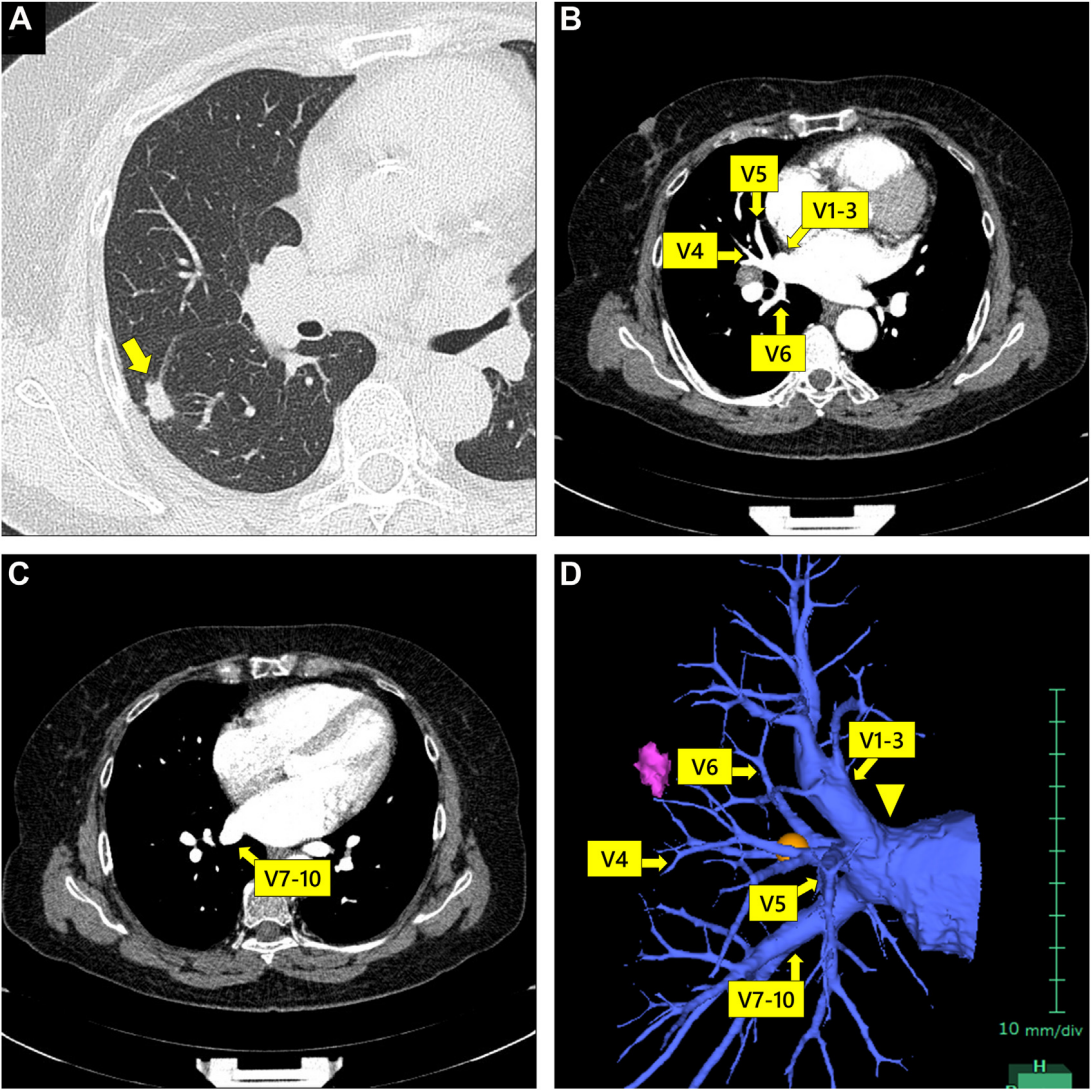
Preoperative computed tomography images. (A) The tumor located at S6 of the right lung (arrow). (B, C) Sequential images demonstrate the common trunk of the right pulmonary veins. (D) Three-dimensional imaging provides stereoscopic visualization of the common trunk of the right pulmonary veins (arrowhead).
